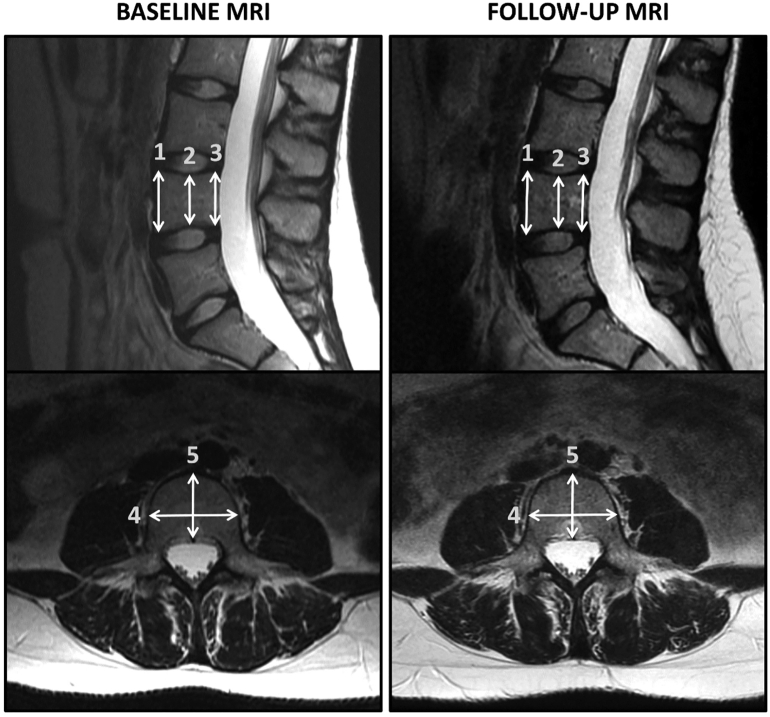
Annotated MR scans of the same individual at age 21 (Baseline MRI) and at age 30 (Follow-up MRI). Above: Midsagittal scans with anterior height (Ruyssen-Witrand et al., 2007), minimum height (International Osteoporosis Foundation, 2017) and posterior height (Bouxsein and Karasik, 2006) measurements marked on L4. Below: Midaxial scans of L4 with annotated width (Odvina et al., 1988) and depth (Heaney et al., 2000) measurements.
